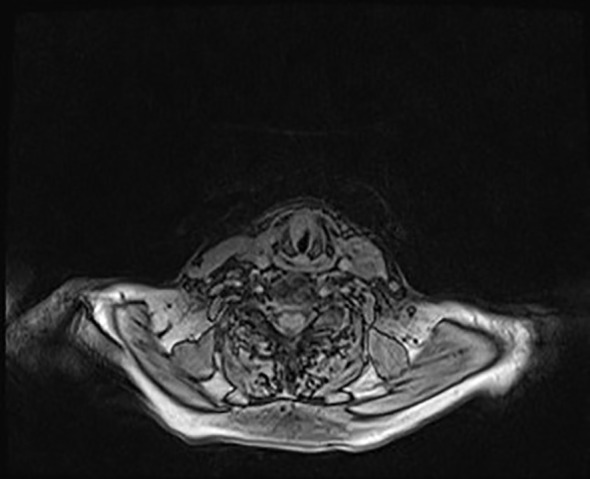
Glottic plane MRI showing the dysplastic hypertrophic neoformation clearly seen at the anterior third of the right true vocal cord while, contralaterally, at the anterior third of the left vocal cord, the neoplasm compatible with granular cell tumor is present, though less viewable.
